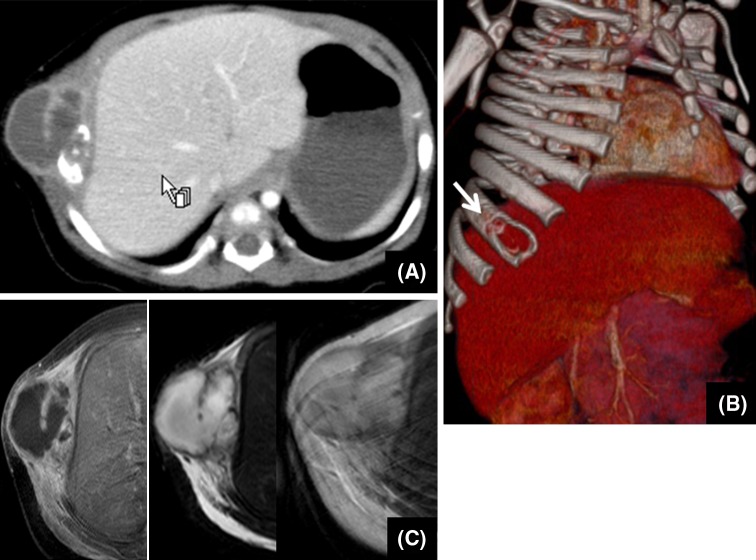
Chest CT demonstrated a multicystic mass, sized 28 × 27 mm and enhanced homogeneously (a). A three-dimensional CT image clarified an osteolytic finding (*←* *arrow*) (b). A MRI revealed an enhanced thickened septum in the tumor (c)
